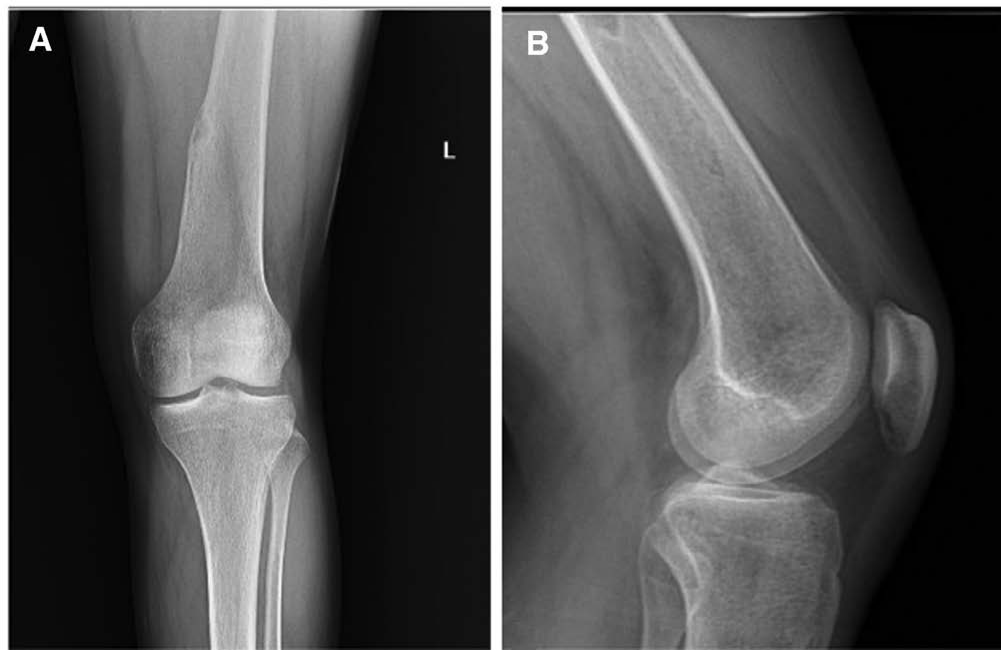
The X-rays of patient No. 1, taken 9 yr after the initial diagnosis, revealed closure of the growth plate, with most of the cartilage tissue having transformed into normal bone by the final follow-up visit at the age of 21 yr old.
